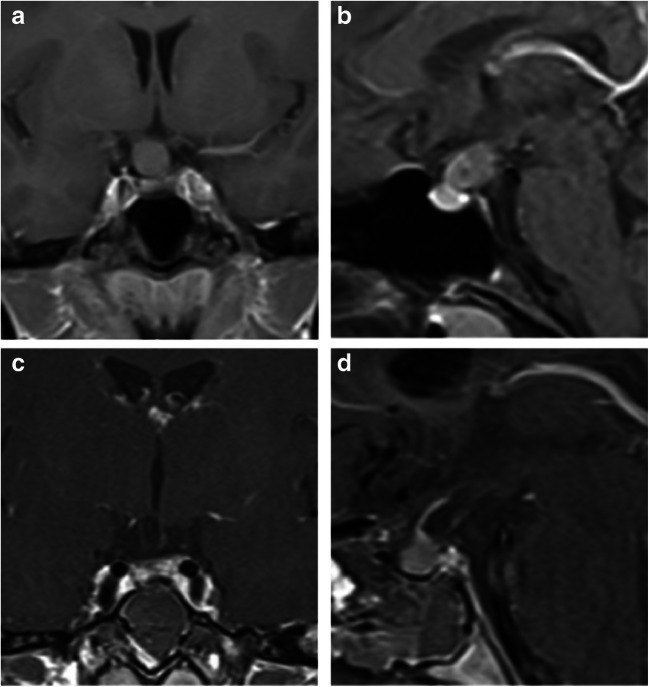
Suprasellar appoach in a Rathke’s cleft cyst in a young female with visual deterioration as a case study. Shown are the preoperative MRI in the coronal plane (a) and sagittal plane (b) and the postoperative MRI in the coronal plane (c) and sagittal plane (d). The cyst content was able to be completely removed with a large fenestration of the cyst’s membrane and no observation of hormonal dysfunction upon further clinical course
